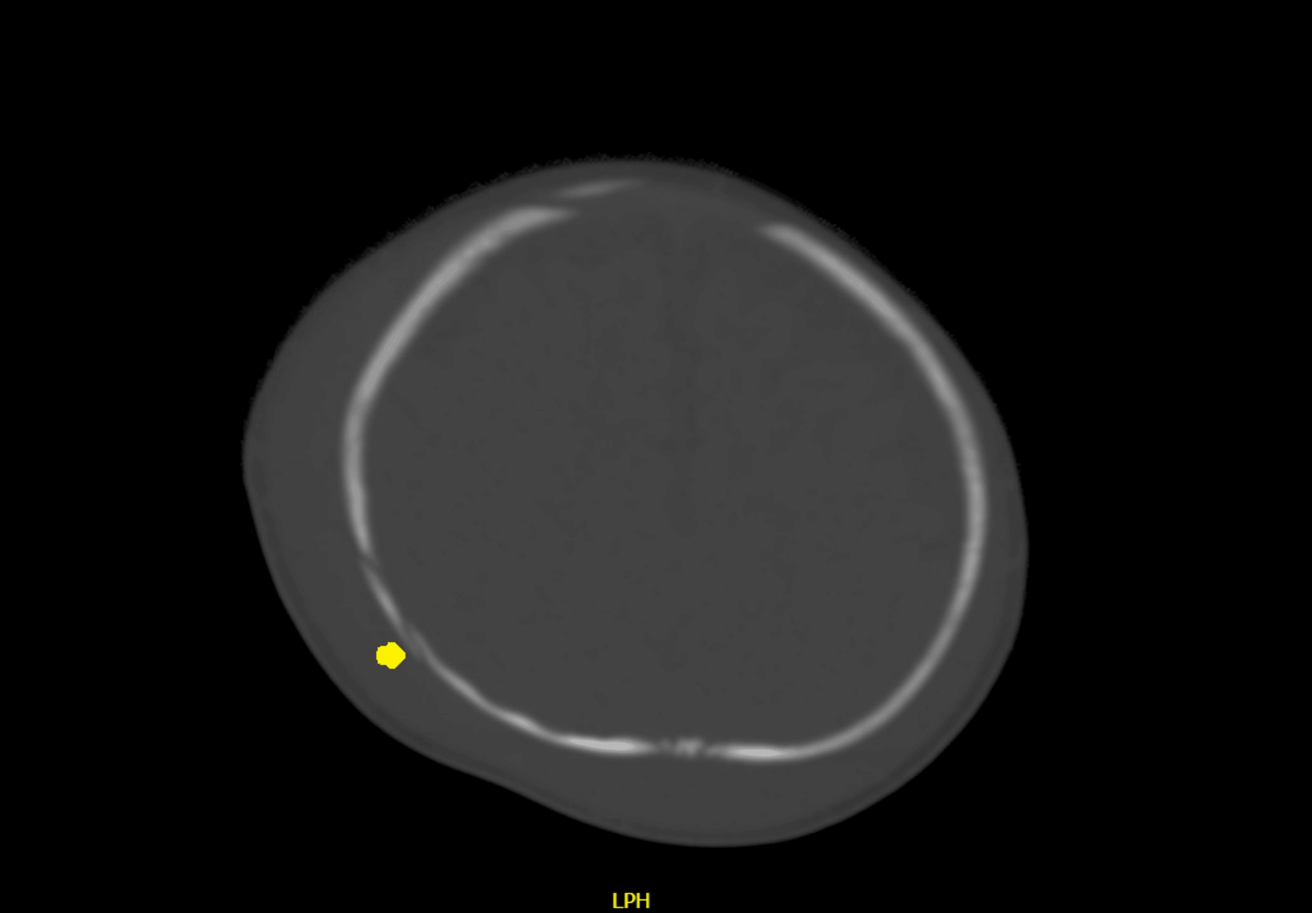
CT of the brain (axial plane) showing fracture of the right parietal bone.
